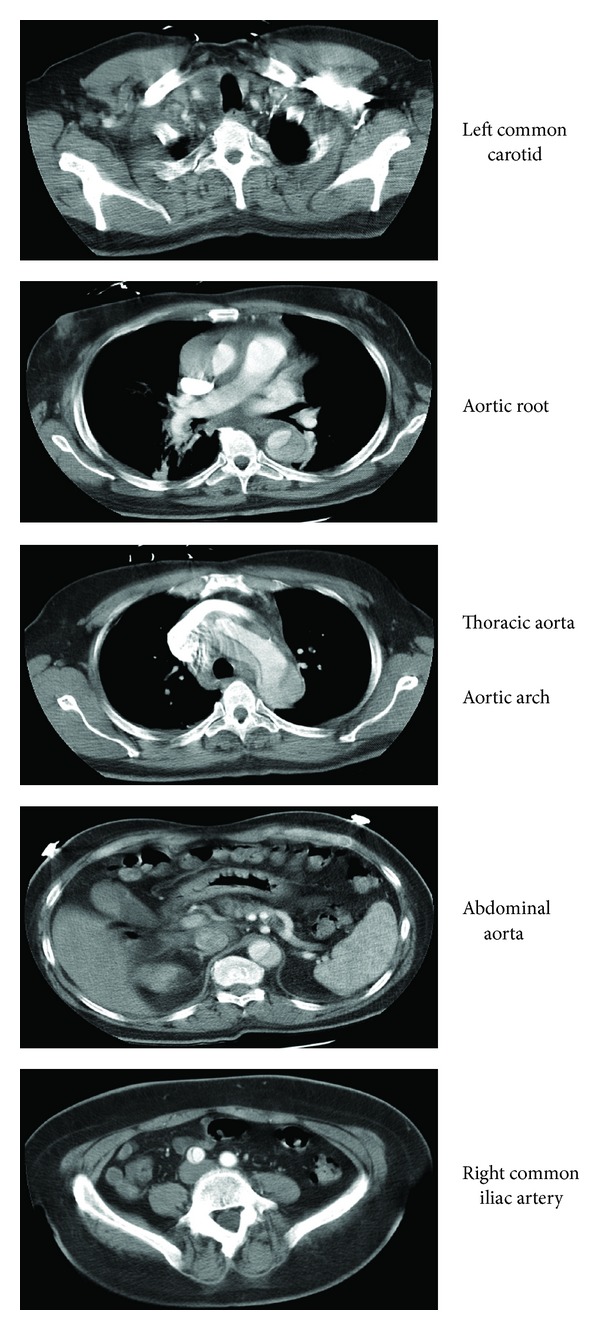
CT angiogram of the chest, abdomen, and pelvis revealed a complete aortic dissection from the aortic ascending upward through the common carotid arteries and downward through the thoracic aorta and abdominal aorta with a final intimal flap in the right common iliac bifurcation.
